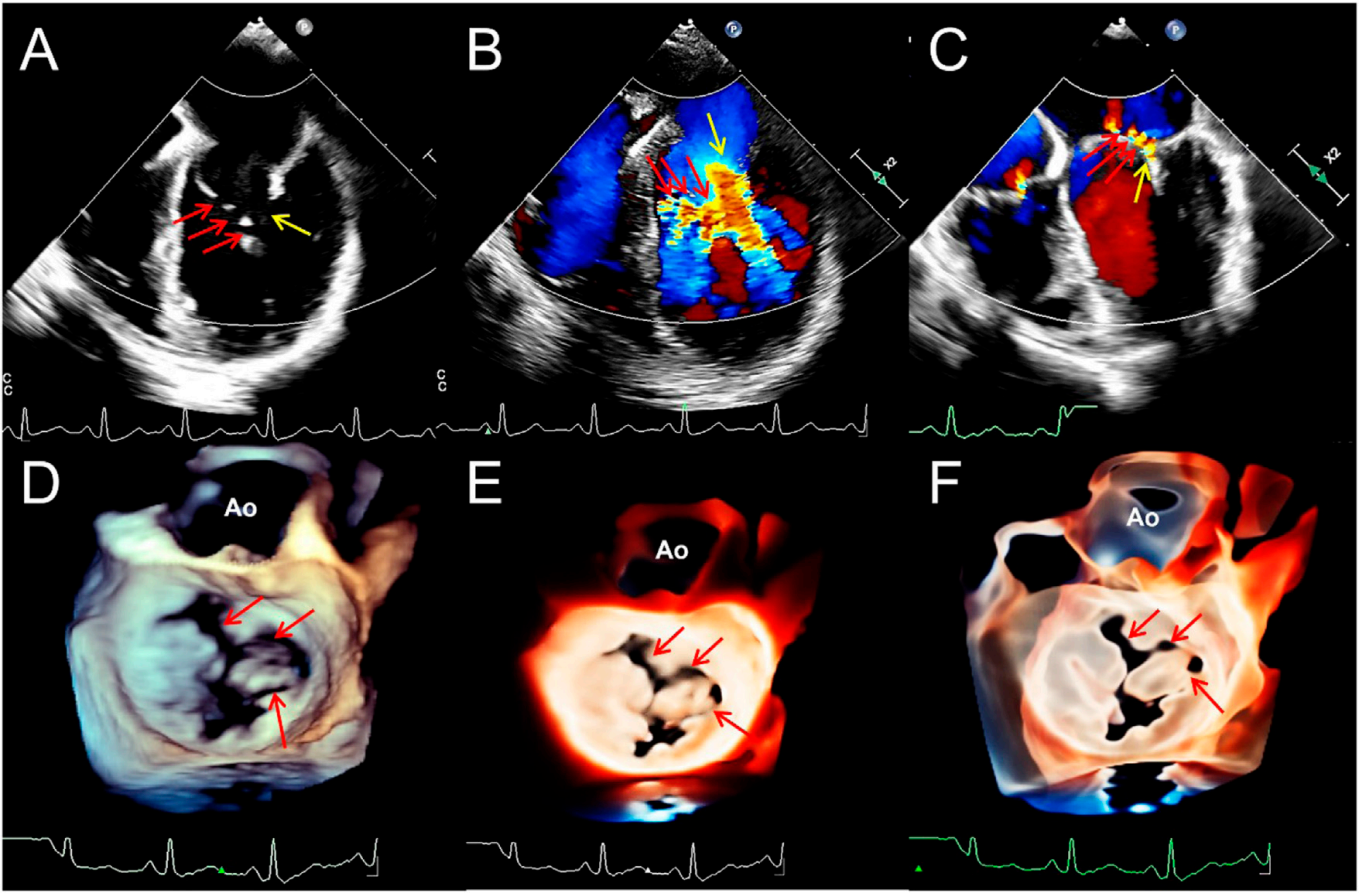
Transesophageal echocardiography reveals multiple mitral valve clefts. (A). 2D echocardiography four-chamber view showing multiple loss of echo in the anterior leaflet of mitral valve (MV) (the sizes of the clefts from A1 to A3 area are 3.1, 4.6, and 3.4 mm respectively, red arrows). The yellow arrow points to the junction between the anterior and posterior leaflets. (B,C). Blood flows into the left ventricle during diastole (red arrows) and regurgitation jets into left atrium during systole (red arrows) from the clefts. Yellow arrows represent the blood flow signals of the normal MV orifices. (D–F). From a surgical perspective, traditional 3D, TrueVue Light, and TrueVue Glass show three irregular clefts (the range of the clefts from A1 to A3 area are 2.5–5.4, 2.5–4.3, and 2.0–3.3 mm, respectively) from the perspective of the left atrium, and all are complete cleft to the annulus. 2D, two-dimensional; 3D, three-dimensional; AO, Aorta; A1, A1 scallop of MV; A3, A3 scallop of MV.
